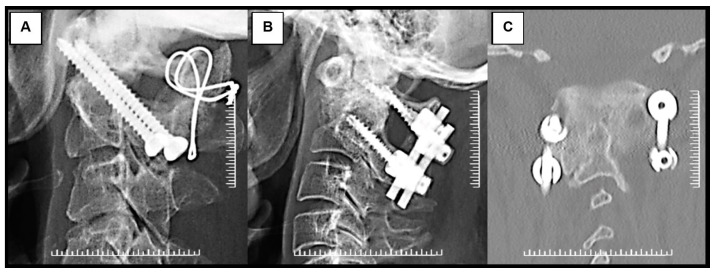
Radiographic images of patients with reducible nonunited type II odontoid fracture with myelopathy treated with (A) Magerl’s C1-C2 transarticular screw fixation technique augmented with supplemental wiring and (B) Harms-Goel C1-C2 screw-rod segmental fixation technique. Coronal CT scan imaging (C) shows C1-C2 complex screw construction with solid fusion mass in Harms-Goel C1-C2 screw-rod segmental fixation technique at 3-month follow-up.
